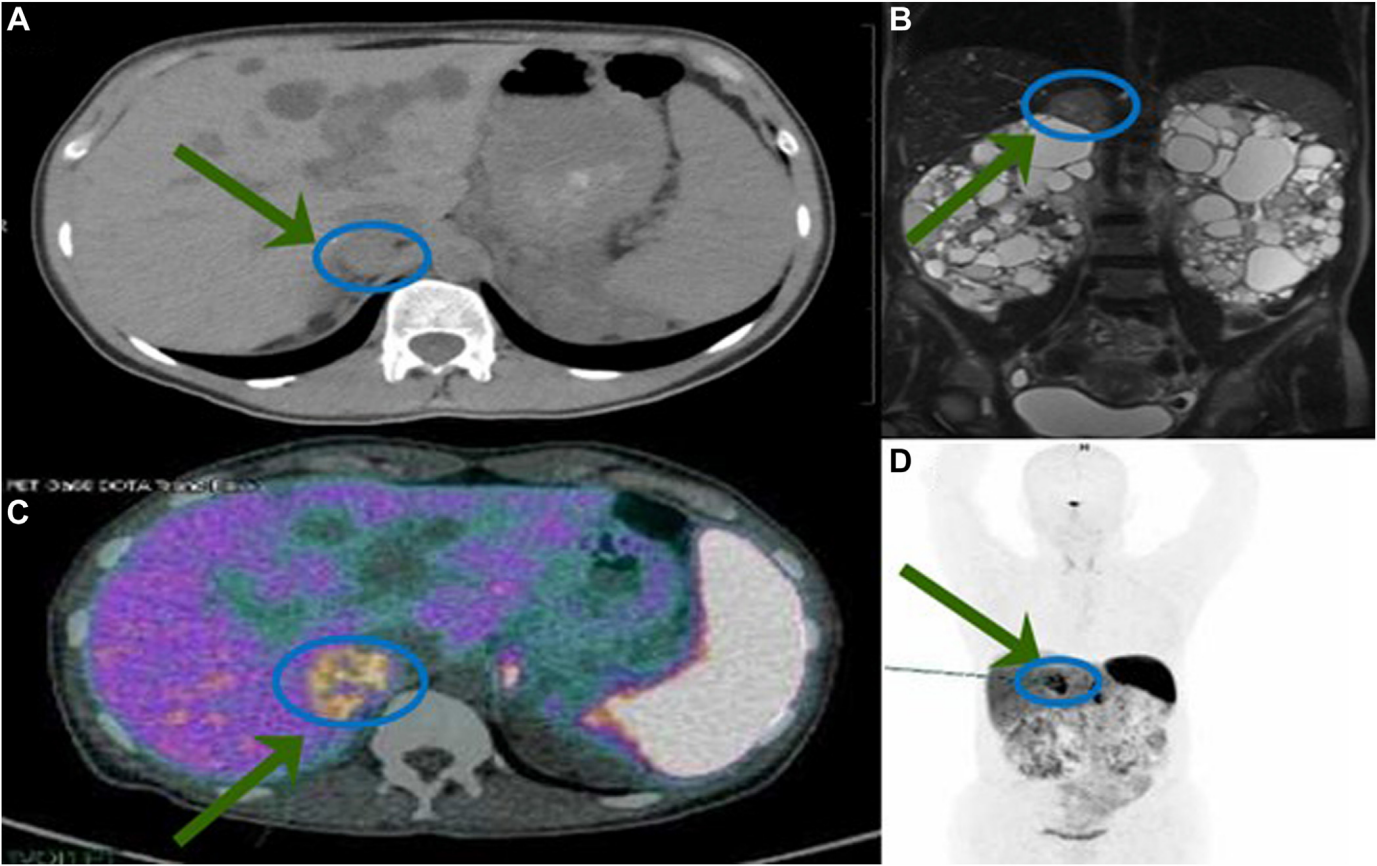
Imaging studies. (*A*) Abdominal computed tomography (CT). An adrenal tumor was present 2 years before diagnosis but overlooked because of the multiple, in part hemorrhagic and closely adjacent cysts in the liver and the right kidney. (*B*) Magnetic resonance imaging (MRI) at diagnosis documenting a 29 mm right adrenal lesion. (*C*, *D*) 86-Ga-DOTATATE positron emission tomography (PET)-CT demonstrating right adrenal uptake without other pathologic lesions.
